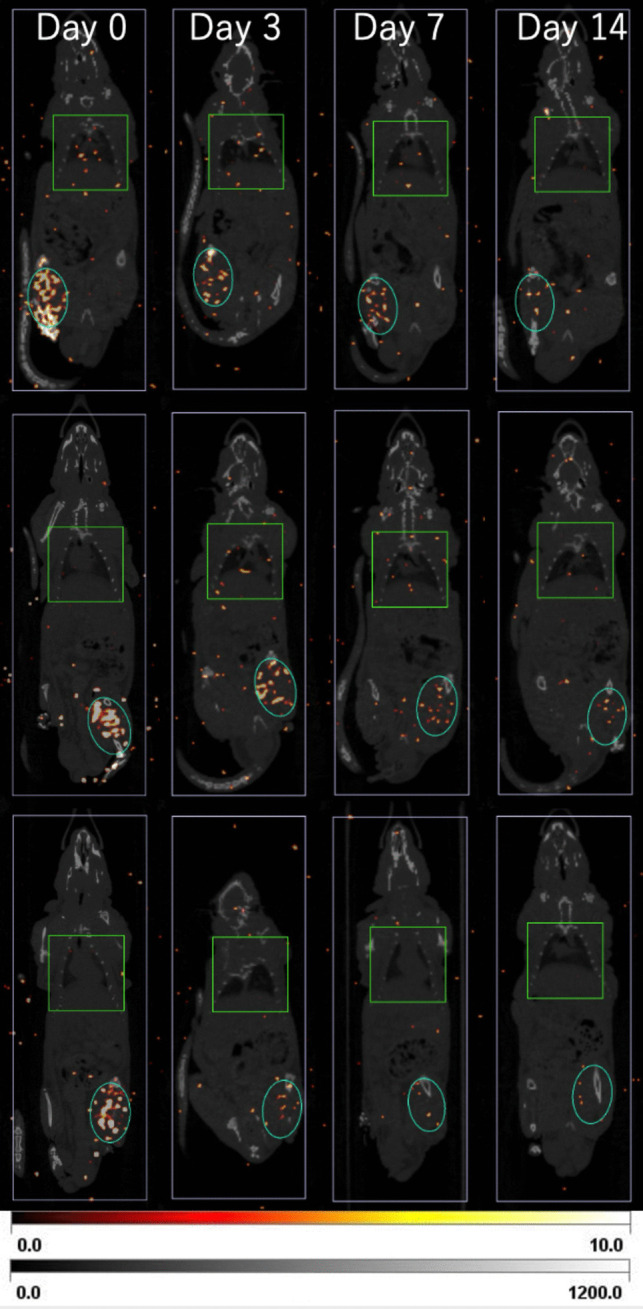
SPECT/CT images showing ^177^Lu-lipiodol biodistribution. The images, featuring ROI_tumor_, ROI_lung_ and ROI_WB_, were acquired on days 0, 3, 7, and 14. High tumor-specific accumulation is evident in the lower leg, while only minimal non-target uptake was observed in other regions, with temporary accumulation in the lungs on day 3
